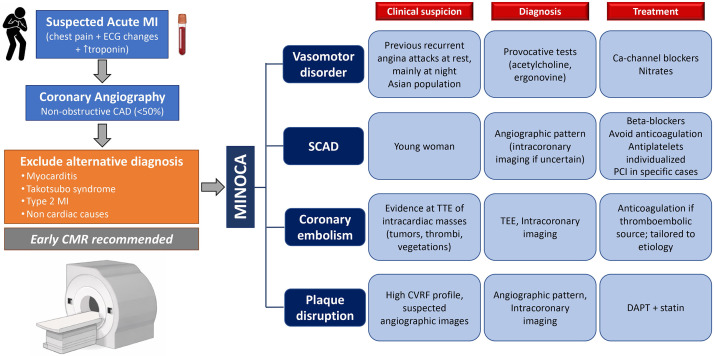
Diagnostic and therapeutic management of patients with myocardial infarction with non-obstructive coronary artery disease (MINOCA). ACEi, angiotensin-converting enzyme inhibitors; CAD, coronary artery disease; CMR, cardiac magnetic resonance; CVRF, cardiovascular risk factors; DAPT, dual antiplatelet therapy; MI, myocardial infarction; PCI, percutaneous coronary intervention; SCAD, spontaneous coronary artery dissection; TEE, transesophageal echocardiogram; TTE, transthoracic echocardiogram.
